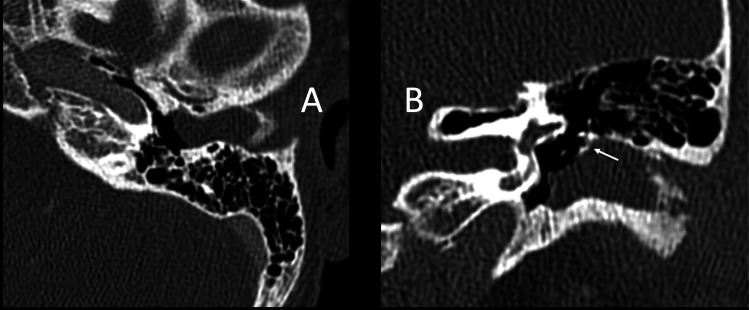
Axial cut (A) and coronal cut (B) of CT scans through the left temporal bone when the patient presented at age 13. Bony hypertrophy and soft tissue obstruction of the left external auditory canal (EAC) are again noted. Cholesteatoma with scutal erosion is noted on the coronal cut (arrow).
